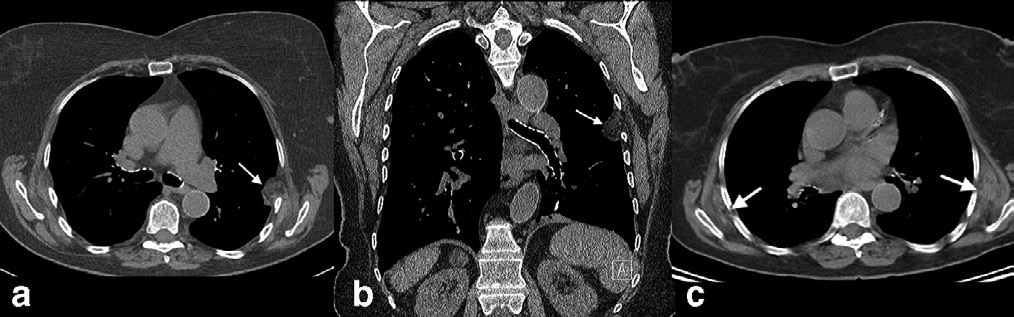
(a, b) Axial and coronal CT images in an 80-year-old female (Case 3) showing a left-sided mixed soft tissue and fatty mass herniating medially through a widened rib interspace at a prior thoracotomy site. Axial CT image in same patient prior to the left thoracotomy, showing bilateral (left greater than right) subscapular chest wall elastofibroma dorsi.
